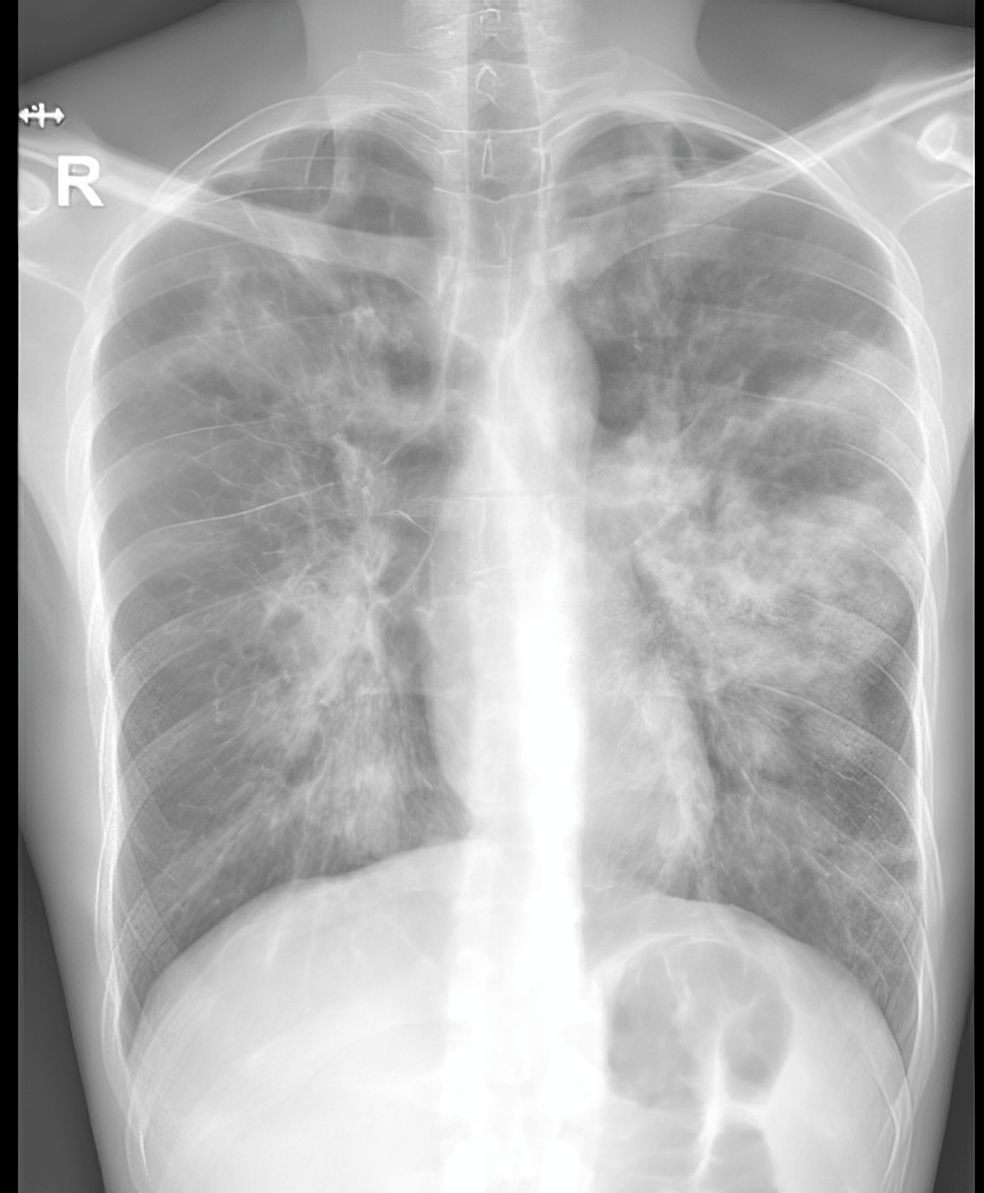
Bilateral infiltrates on initial chest X-ray
